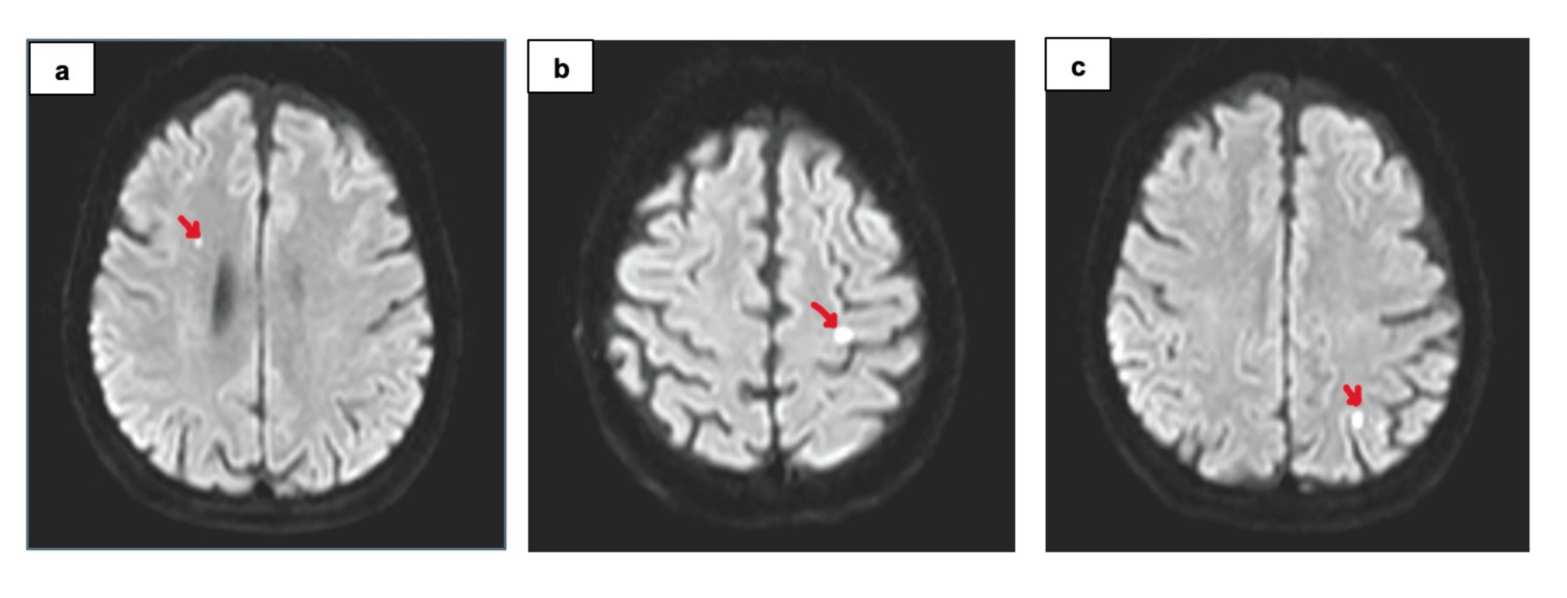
Follow-up DWI revealed multiple small, nodular foci of diffusion restriction consistent with acute infarctions a-c: These lesions  were localized in the left occipital and temporal lobes, right frontal lobe, as well as the left parietal and left frontal lobes (red arrows) DWI: Diffusion-weighted imaging
